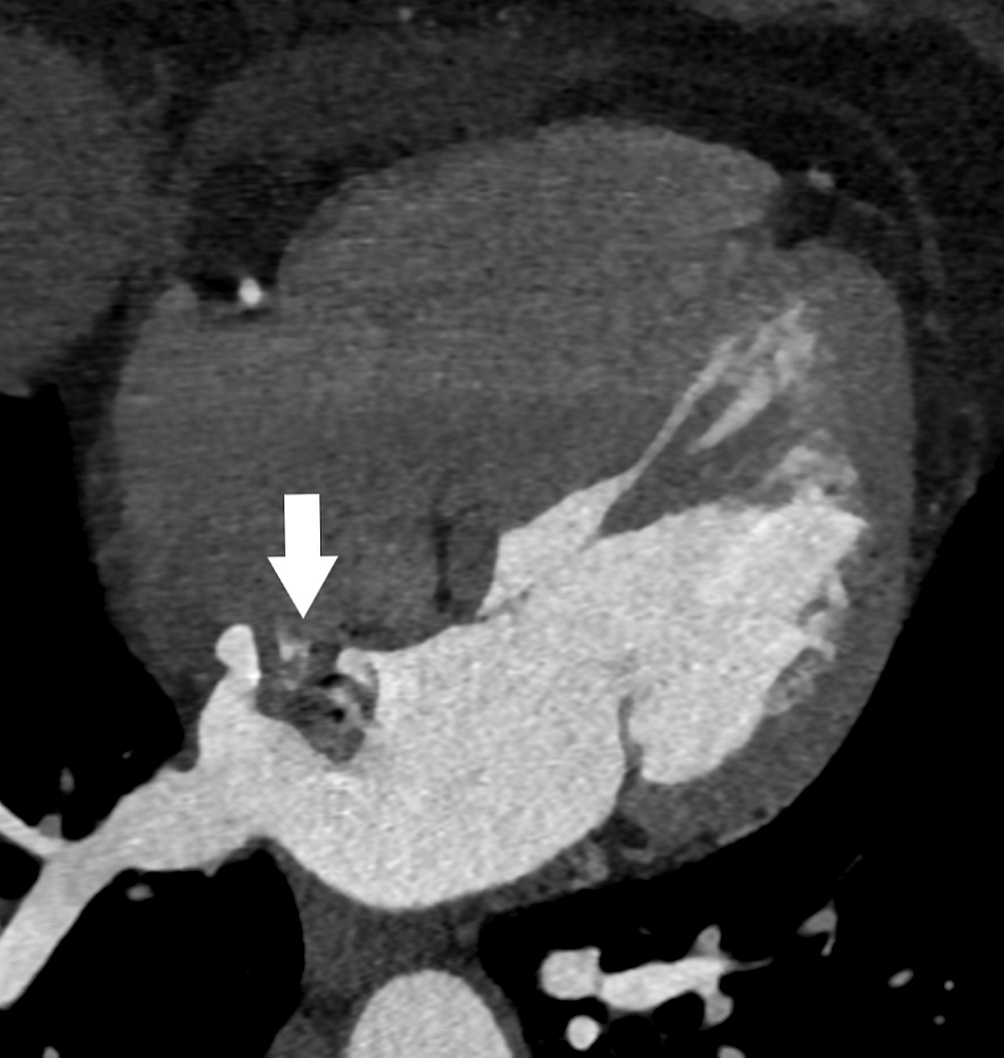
Cardiac CT of a left atrial myxoma with a wide stalk (arrow)
